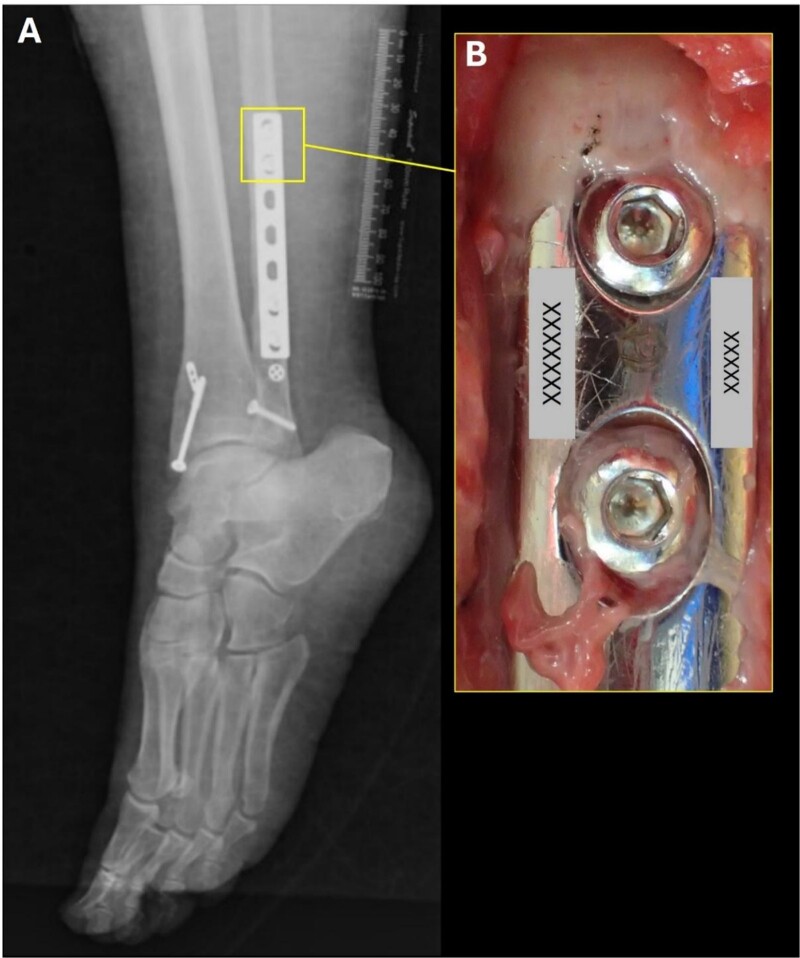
Postmortem radiographs of left distal lower limb, where the surgical plate fixed with screws and surgical ligament anchors can be observed (A). Detail photograph of the superior part of the surgical plate that showed the identification numbers indicated with “X” (B).
